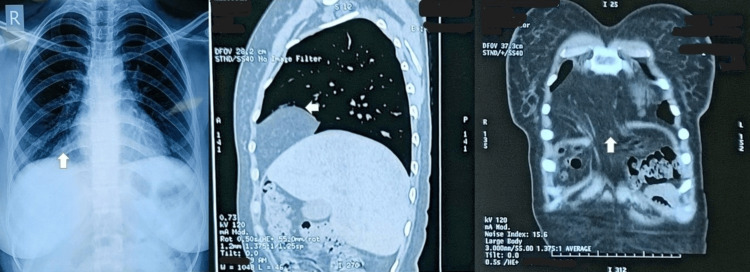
Chest roentgenogram (posteroanterior view) suggestive of nonspecific opacity at the right cardiophrenic angle (left) (white arrow). CT thorax (middle and right) confirming anteromedial diaphragmatic defect with OF herniation without bowel loops (white arrow). CT: computed tomography; OF: omental fat.
